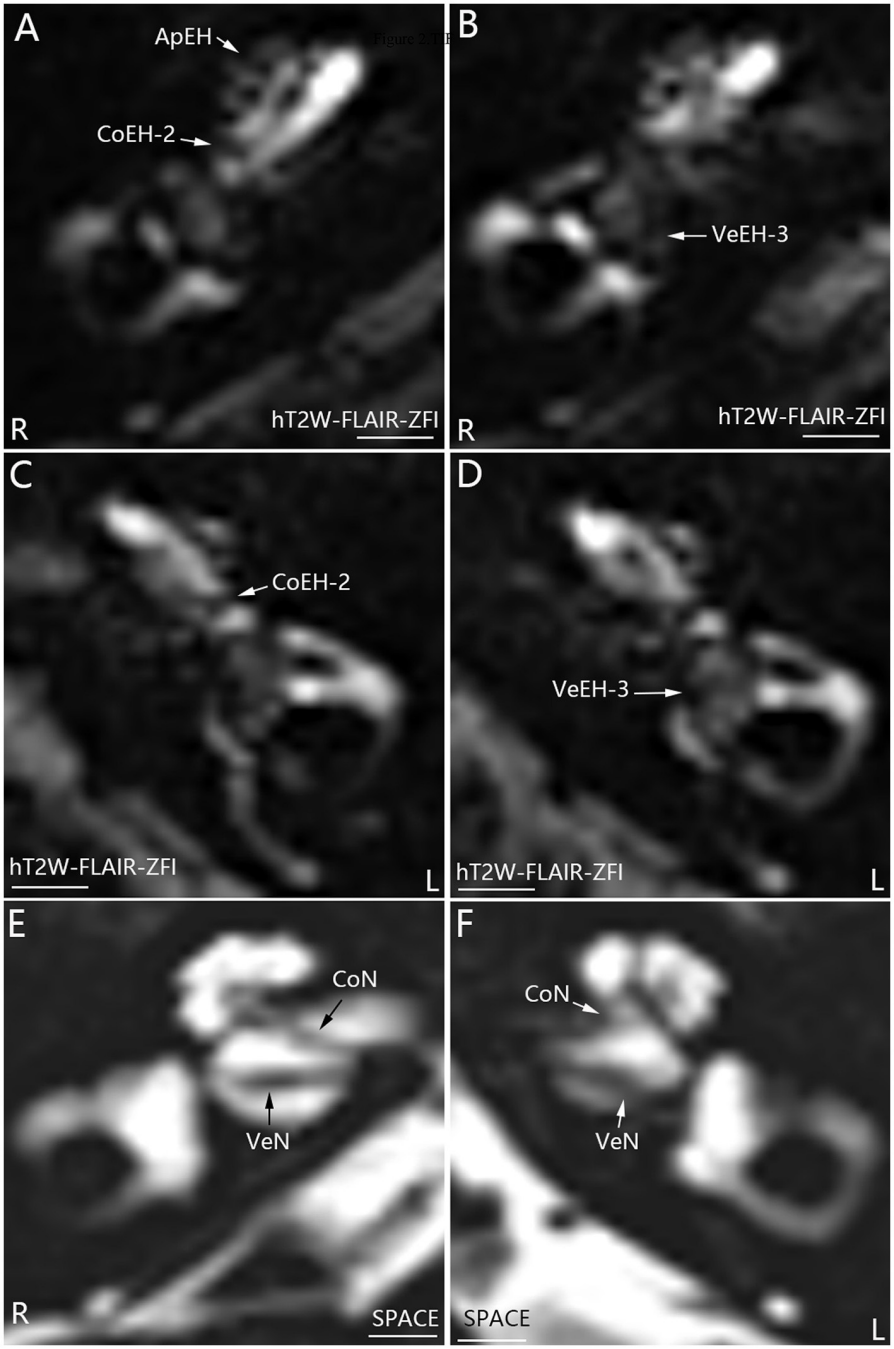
Representative MRI demonstrating EH in both ears in patient *M* with definite bilateral MD (A–D). MR images were taken using the hT_2_W-FLAIR-ZFI sequence 24 h after intratympanic injection of 0.1 ml of 20-fold-diluted Gd-DTPA on both sides. Apical EH (ApEH) was also observed in the right ear (R) (A) but not in the left ear (L) (C). Neither inner ear fibrosis nor vestibular schwannoma was observed on either side imaged using the SPACE sequence (E, F). CoEH-2, grade 2 cochlear EH; CoN, cochlear nerve; VeEH-3, grade 3 vestibular EH; VeN, vestibular nerve. Scale bar = 3.0 mm.
